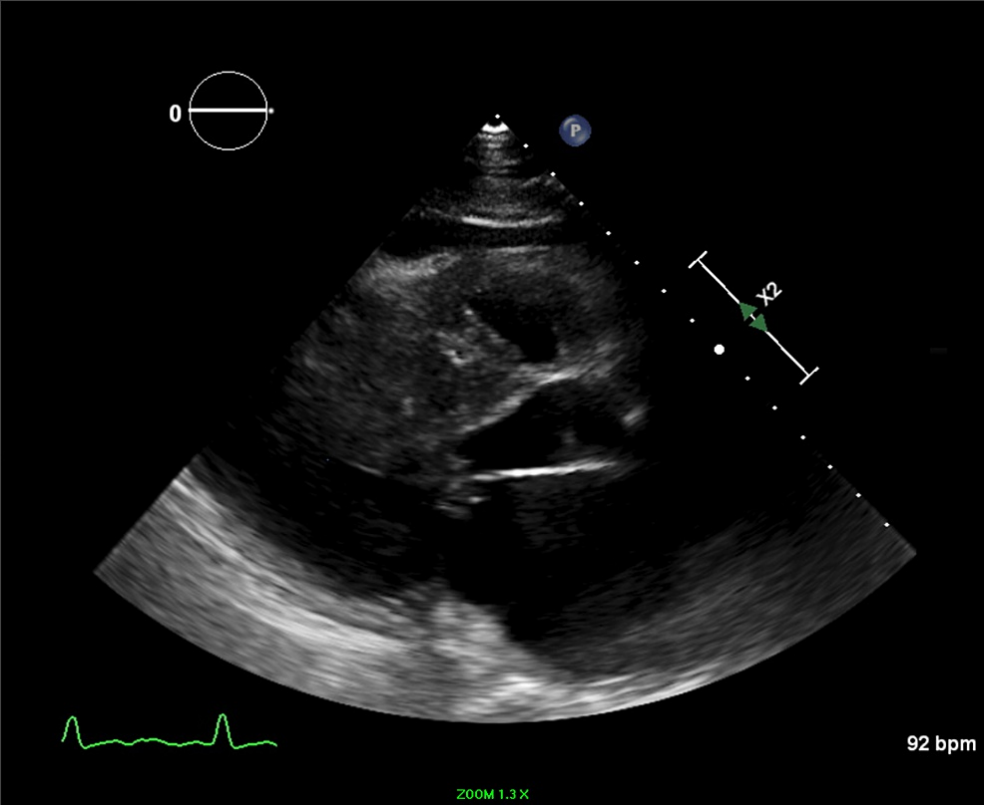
TTE parasternal long axis view TTE: Transthoracic echocardiogram Parasternal long axis view shows chordal systolic anterior motion and thickened anterior mitral valve leaflet.
